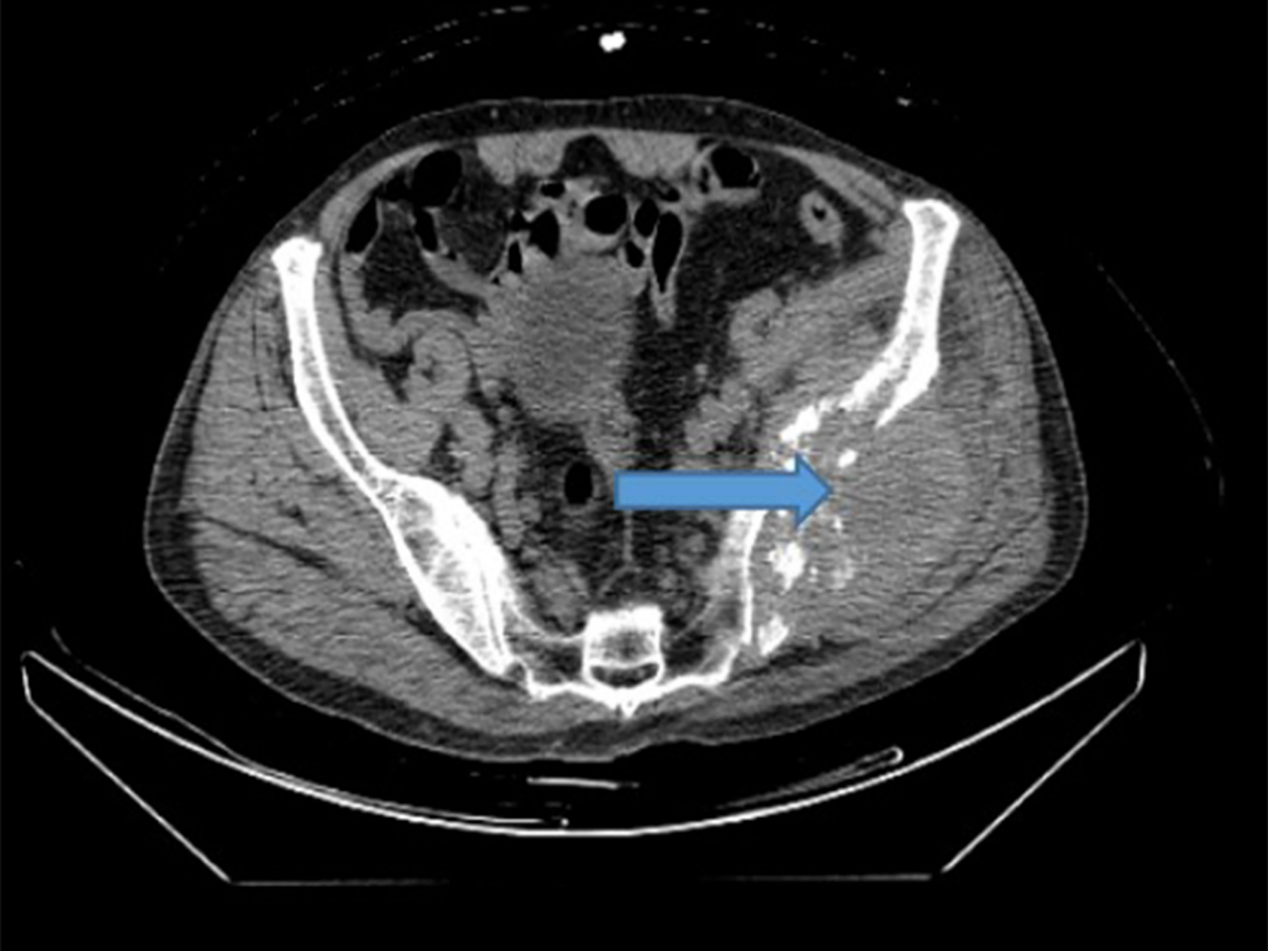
Preoperative SPECT/CT showed bone destruction in the corresponding part of the left iliac crest and soft tissue mass in the left iliac crest lesion, which was considered as bone metastasis.
